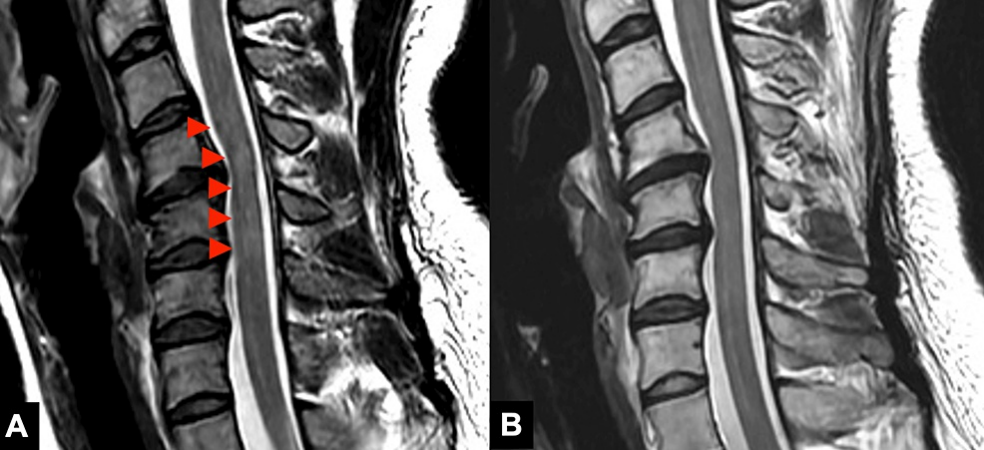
Cervical MRI T2-weighted images before and after steroid pulse therapy Pretreatment images showed T2-weighted high signal at the level of cervical vertebral bodies 4-5 (A), which resolved slowly three months after treatment (B).
